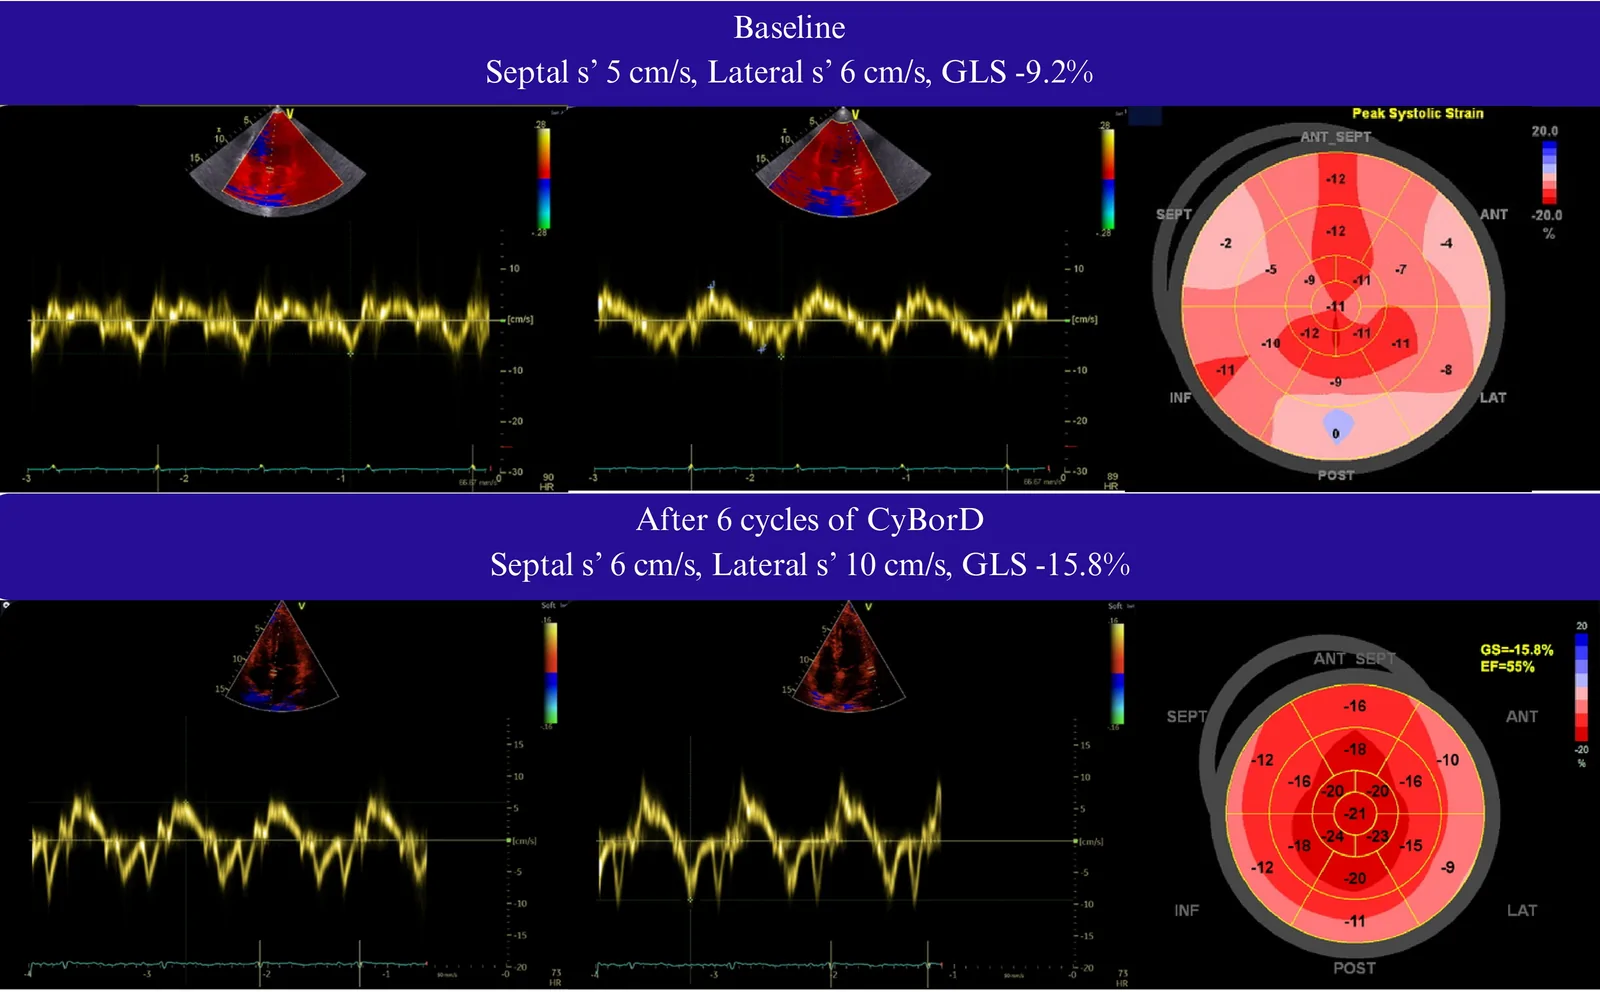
Serial Improvement in Tissue Doppler Velocities and GLS After Chemotherapy for Immunoglobulin Light Chain Cardiac Amyloidosis Baseline echocardiography (top panels) showed impaired longitudinal systolic function with reduced septal and lateral mitral annular tissue Doppler systolic velocities (s′ 5 cm/s and 6 cm/s, respectively) and severely abnormal global longitudinal strain (GLS) (−9.2%). Following 6 cycles of cyclophosphamide, bortezomib, and dexamethasone (CyBorD) (bottom panels), there was interval improvement in annular systolic velocities (septal s′ 6 cm/s, lateral s′ 10 cm/s) and GLS (−15.8%), reflecting early functional myocardial recovery after therapy. CyBorD = cyclophosphamide, bortezomib, and dexamethasone; GLS = global longitudinal strain.
